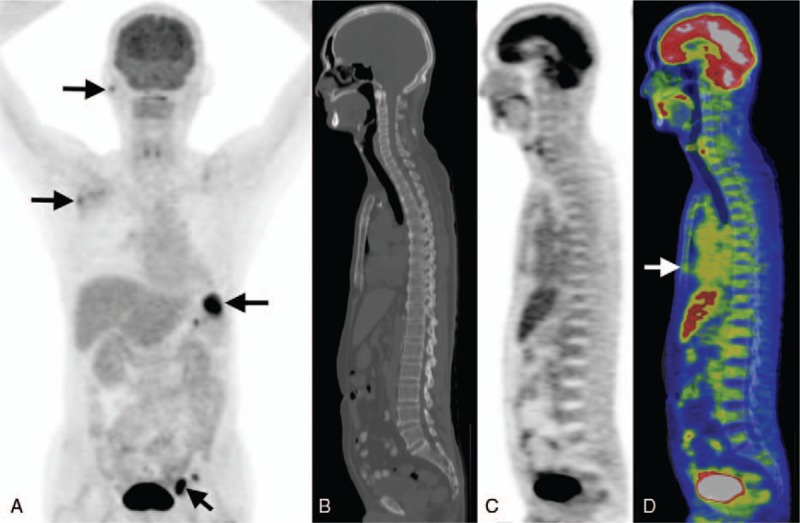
Demonstration of the FDG PET/CT image and the SUV measurement for FDG uptake of bone marrow. This 69-year-old man was diagnosed with stage III DLBCL. Maximal intensity projection (MIP) of the pretreatment FDG PET revealed multiple high grade FDG-avid lesions in the right parotid, right axillary, left inguinal regions, as well as the spleen (arrows in A). Using CT image for localization, the maximal SUVst was recorded by placing the ROIs over the sternum slice by slice (arrow in D). His maximal SUVt and SUVst were 15.1 and 1.78, respectively. Thereafter, he received treatment with R-CHOP regimen. However, the patient expired 10 months after diagnosis due to progression of the disease. A = MIP, B = CT, C = PET, D = fused PET and CT images.
